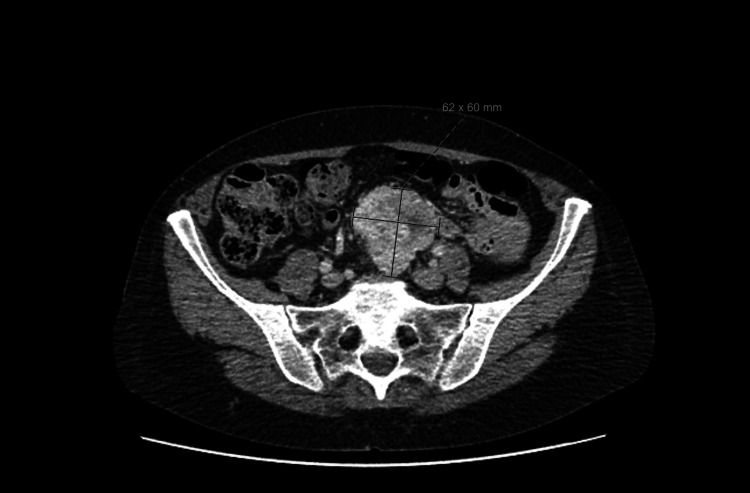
CT Scan showing 6 x 6 x 5.5cm presacral mass which appears to be small bowel in origin
